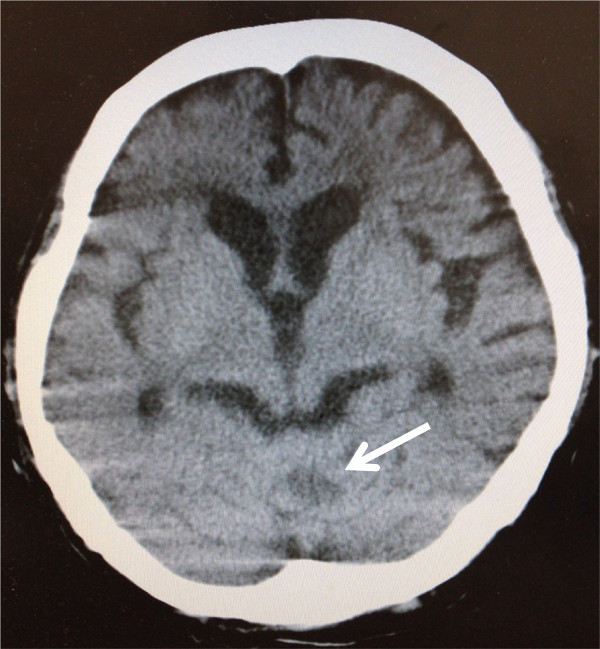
At 10 weeks after radiotherapy, CT shows a tumor reduced in size (arrow).
